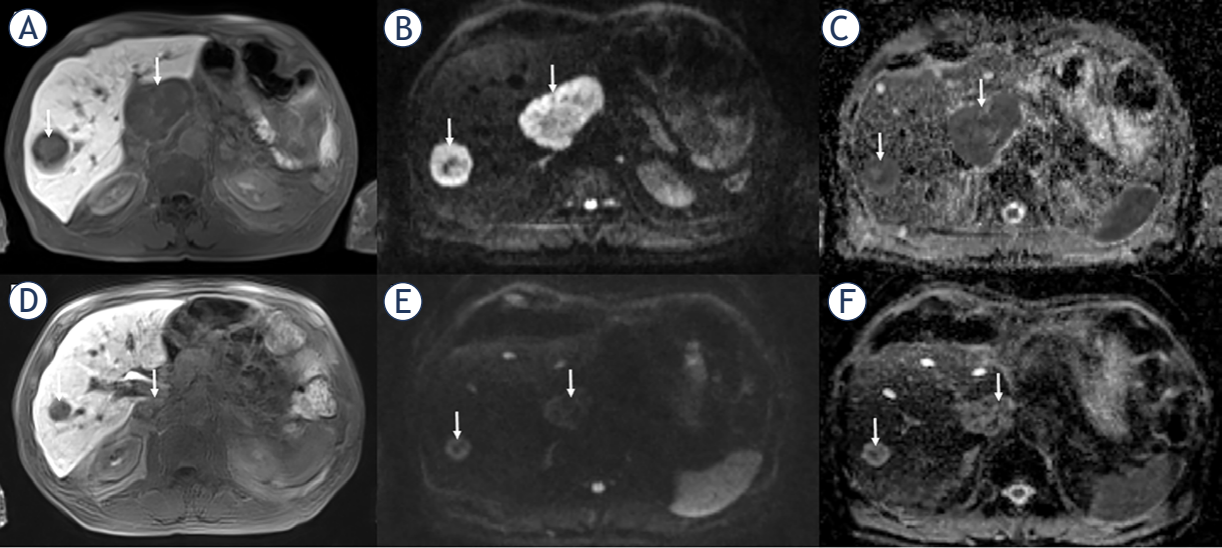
A 72-year-old man with liver metastasis of pancreatic NET classified as responder with a PFS of 38 months. The baseline axial contrast-enhanced T1- weighted image (hepatobiliary phase) (A) shows hypointense lesions (arrows) in segment 8 and exophytic in segment 1. The metastases show (B) restricted diffusion (arrows) with high signal on axial DW-MR image b = 800 s/mm^2^ and dark signal (arrows) on ADC map (C). After initiation of CAPTEM, the metastases (arrows) exhibited a decrease in size (D) On the axial DW-MR image b = 800 s/mm^2^, the metastasis (arrow) (E) demonstrated less hyperintense signal to liver and predominantly hyperintense signal (circle) on the ADC map (F) indicating less restricted diffusion compared to the preinterventional image. ADC = apparent diffusion coefficient; CAPTEM = capecitabine and temozolomide; DW-MR = diffusion-weighted magnetic resonance; NET = neuroendocrine tumor; PFS = progression-free survival; PR =partial remission; TARE = transarterial radioembolization
